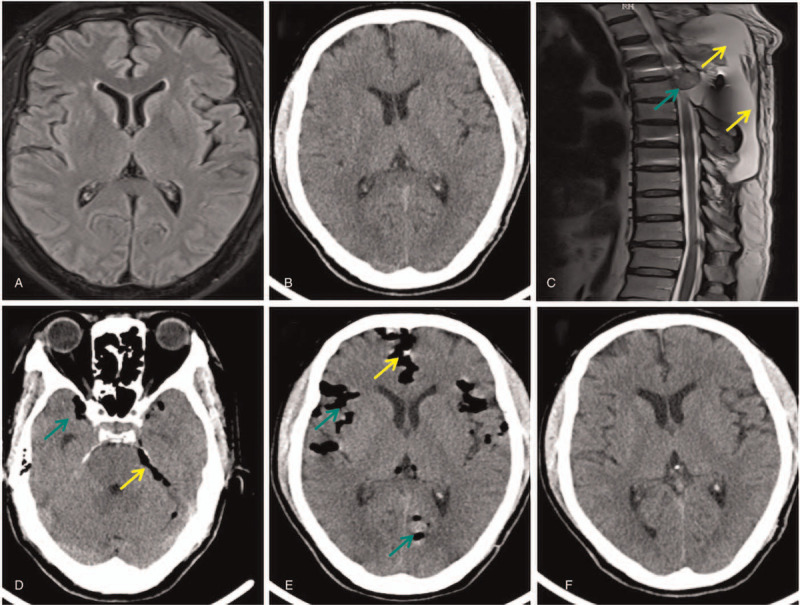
Neuroimaging results before and after the occurrence of pneumocephalus. Preoperative brain magnetic resonance imaging (MRI, A) and computed tomography (CT, B) showing no obvious abnormality. MRI of thoracic vertebra (C) showing roundish nodules (green arrow) in the right foramen intervertebral region of T4–5, which are recurrent or residual tumors, and subcutaneous hydrops (yellow arrows) in the dorsal side of T3–8 vertebrae. Brain CT 16 days after surgery showing massive air densities in the lateral fissure (D and E, green arrows), circle cistern (D, yellow arrow), and anterior longitudinal fissure (E, yellow arrows). Repeated head CT 5 days after pneumocephalus showing complete resolution of the pneumocephalus (F).
